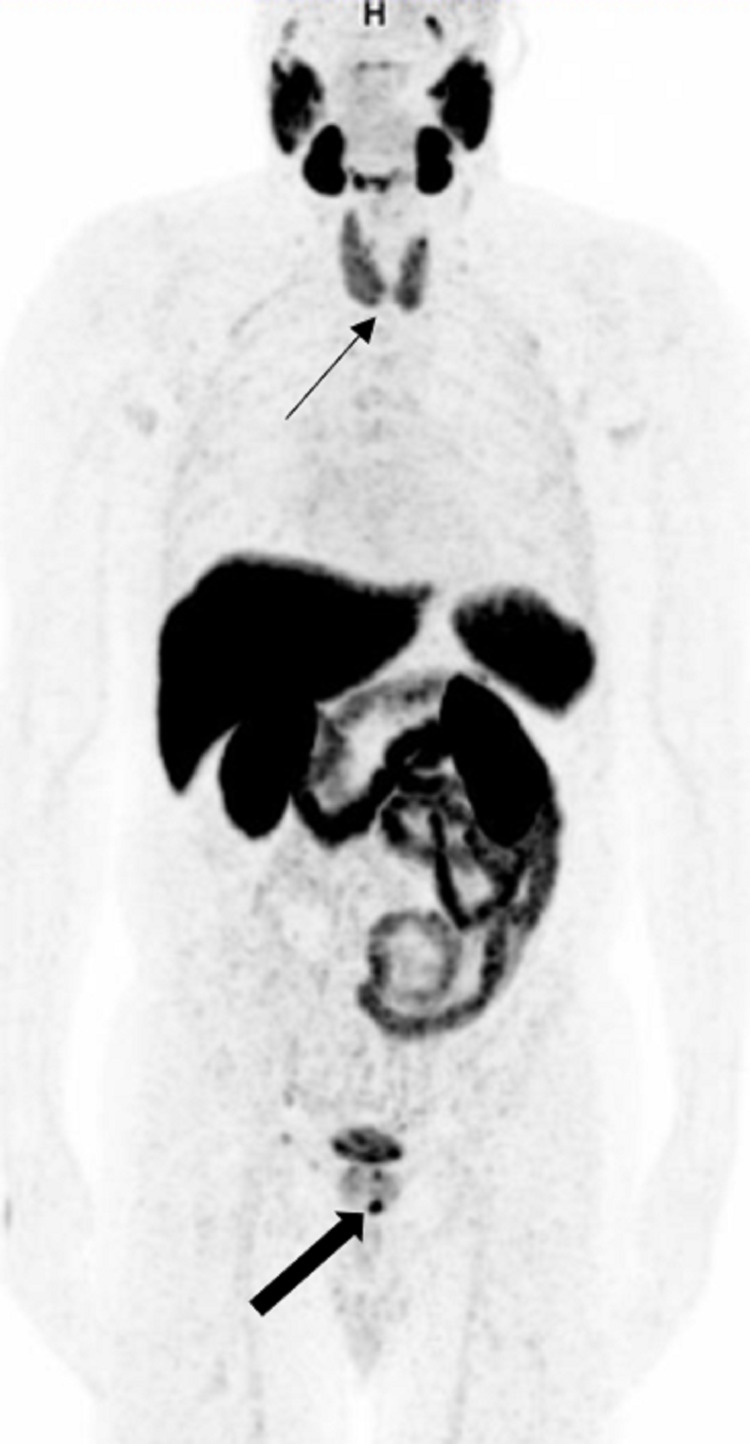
Thyroiditis 68Ga PSMA PET MIP image shows diffuse moderate uptake throughout the bilateral lobes of the thyroid (black thin arrow) likely due to thyroiditis. Note the heterogeneous moderate to high PSMA uptake in the prostate gland involving both lobes with the highest focal uptake on the left (black thick arrow) in this patient with newly diagnosed prostate cancer with PSA level 12.
